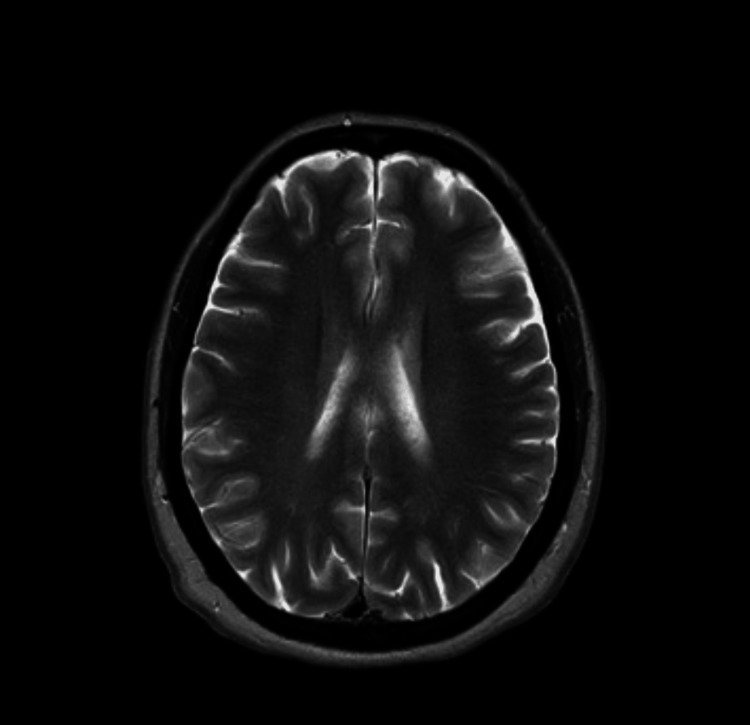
Normal MRI scan of the brain A brain MRI was done to rule out encephalitis and SAH. SAH: Subarachnoid hemorrhage.
